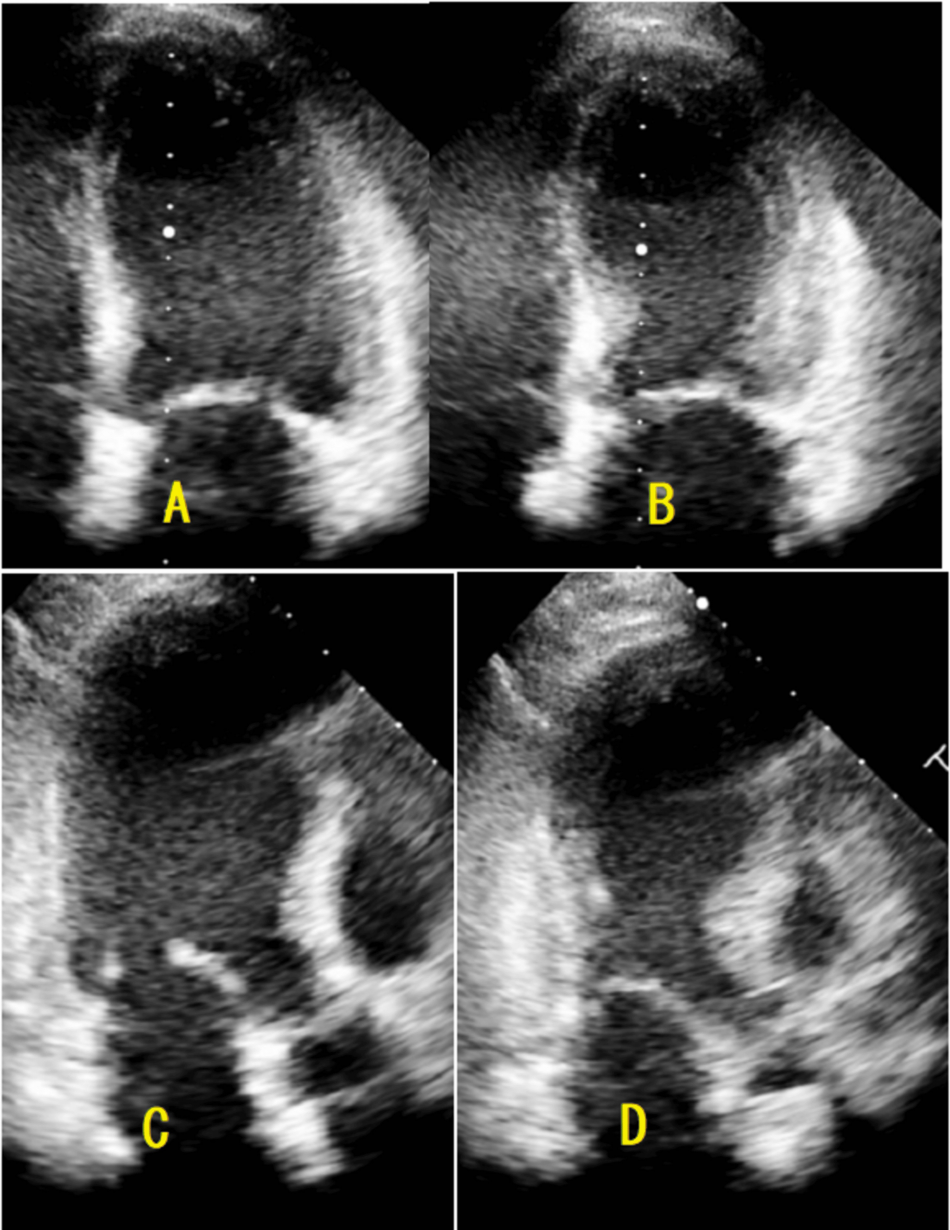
Echocardiographic images showing apical four-chamber and three-chamber views of the patient, highlighting classic apical ballooning with akinesia and hyperdynamic basal segments Apical four-chamber view in diastole (A) and systole (B) and three-chamber view in diastole (C) and systole (D).
